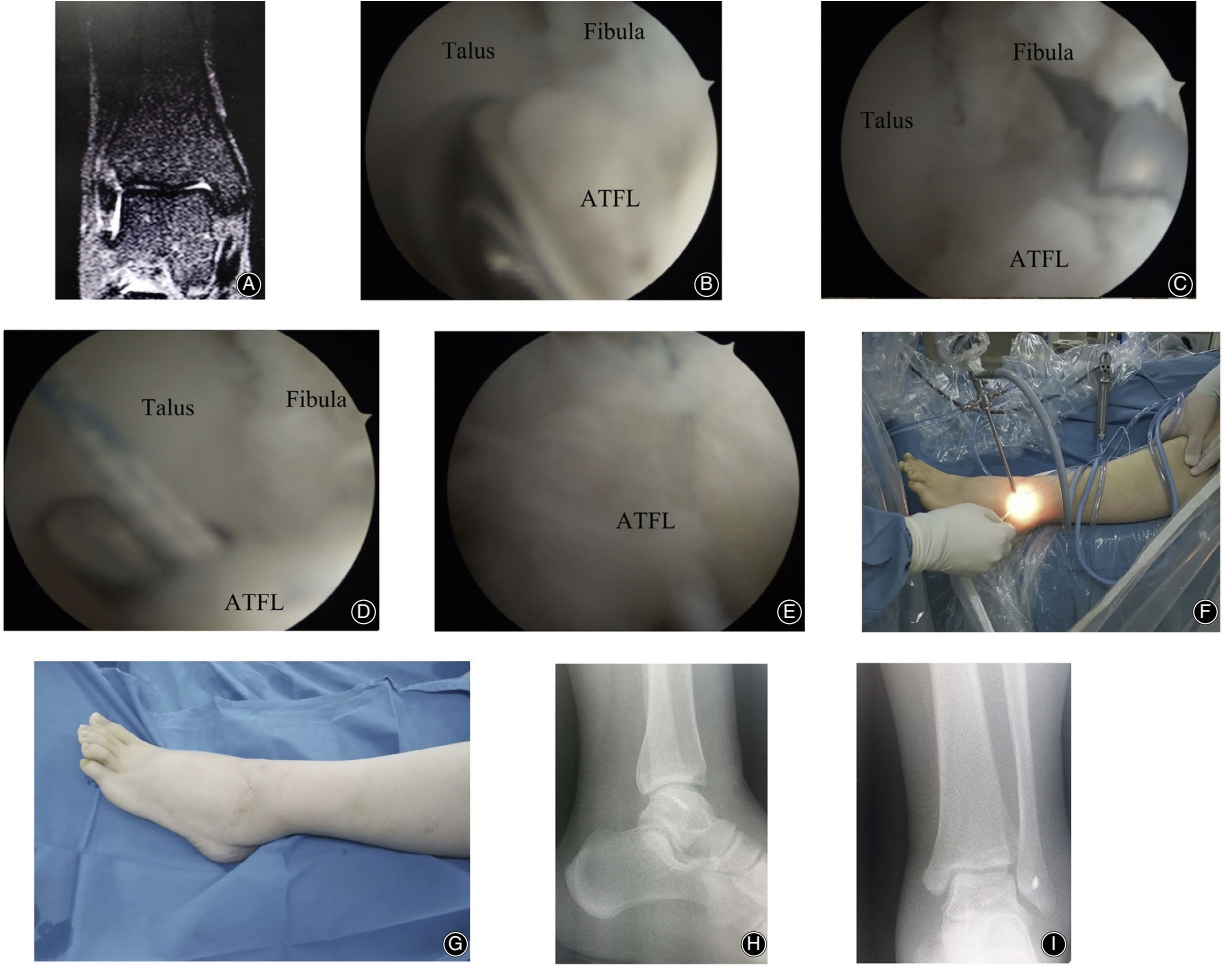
A 28‐year‐old female. The symptoms of CLAI were still presented after 8 months of conservative treatment. (A) Preoperative coronal MRI scan of the left ankle suggested the interruption of ATFL. (B) Flaccid and tension‐free ATFL that avulsed from the fibula point was observed under the arthroscopy. (C) A double wire anchor with a diameter of 3.5 mm was inserted into the fibular footprint region. (D) The ATFL was sutured with a suture hook under arthroscopy. (E) The ATFL was sutured and it returned to normal tension under arthroscopy. (F) External image of the arthroscopic procedure during the operation. (G) The portals of the surgery after the operation. (H) Lateral X‐ray of the ankle after surgery. (I) Anterior–posterior X‐ray of the ankle after surgery.
